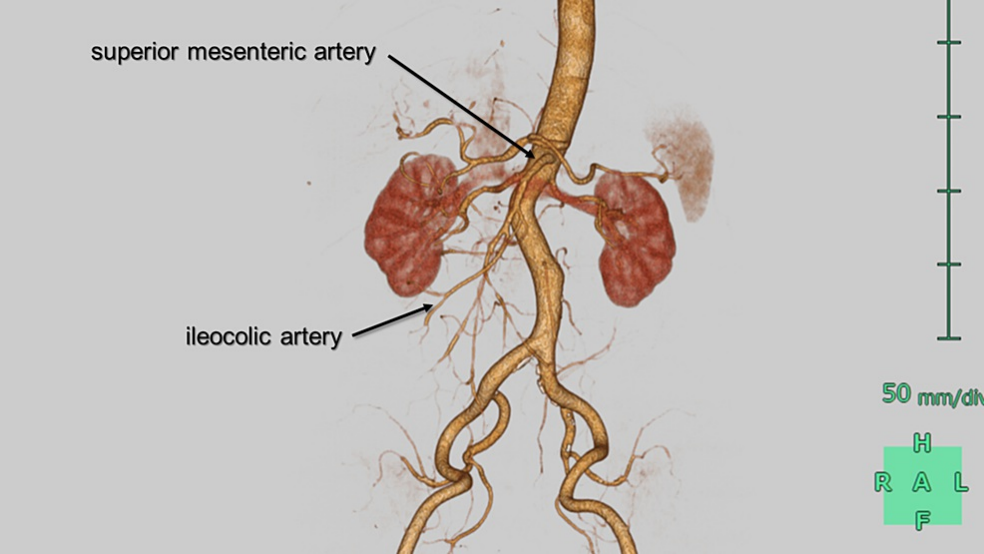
3D computed tomography of superior mesenteric artery Peripheral branches are weakly enhanced.
